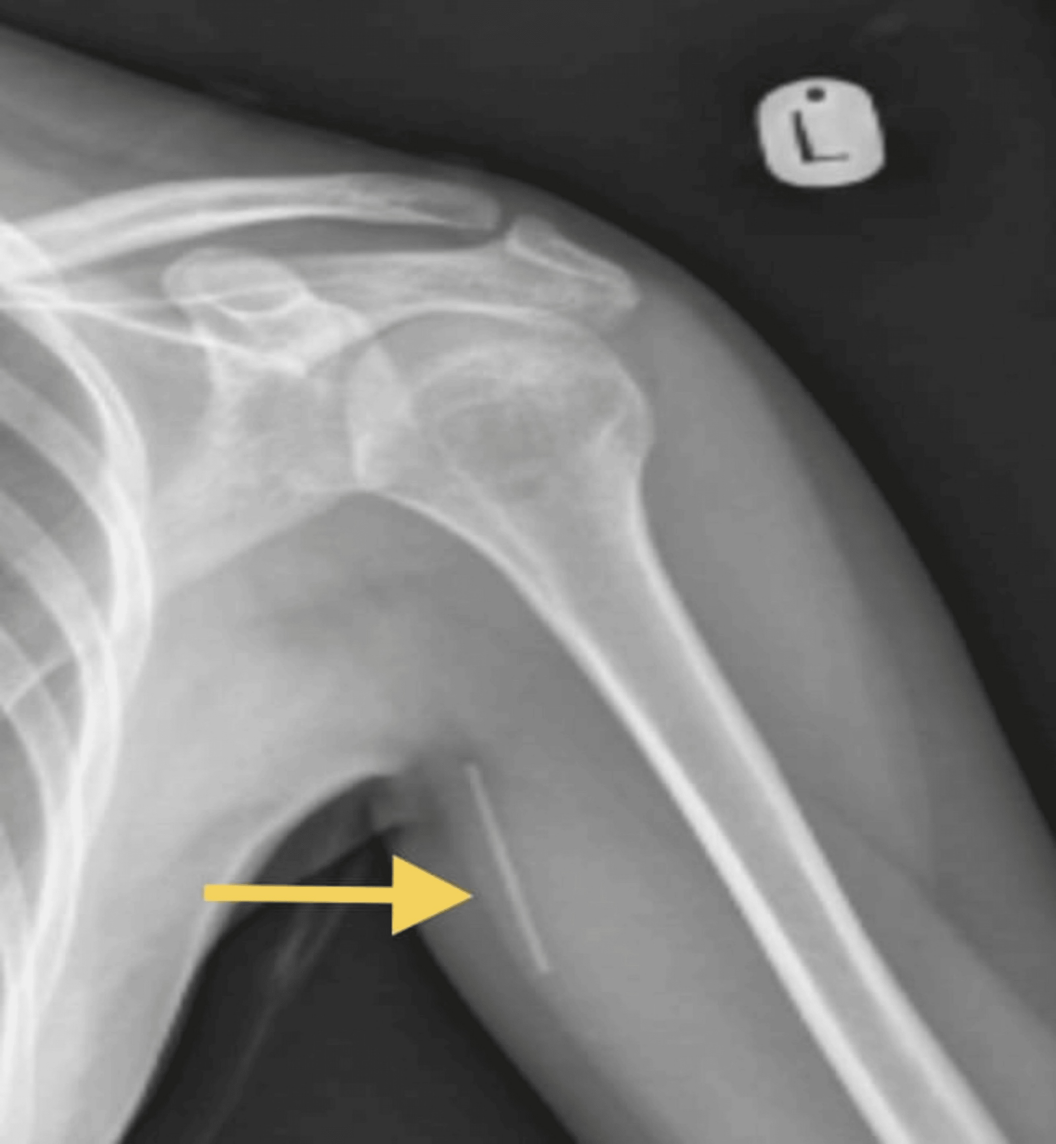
X-ray of the upper extremity showing a linear radiopaque structure in the left upper arm soft tissue, consistent with the characteristics of an implant
